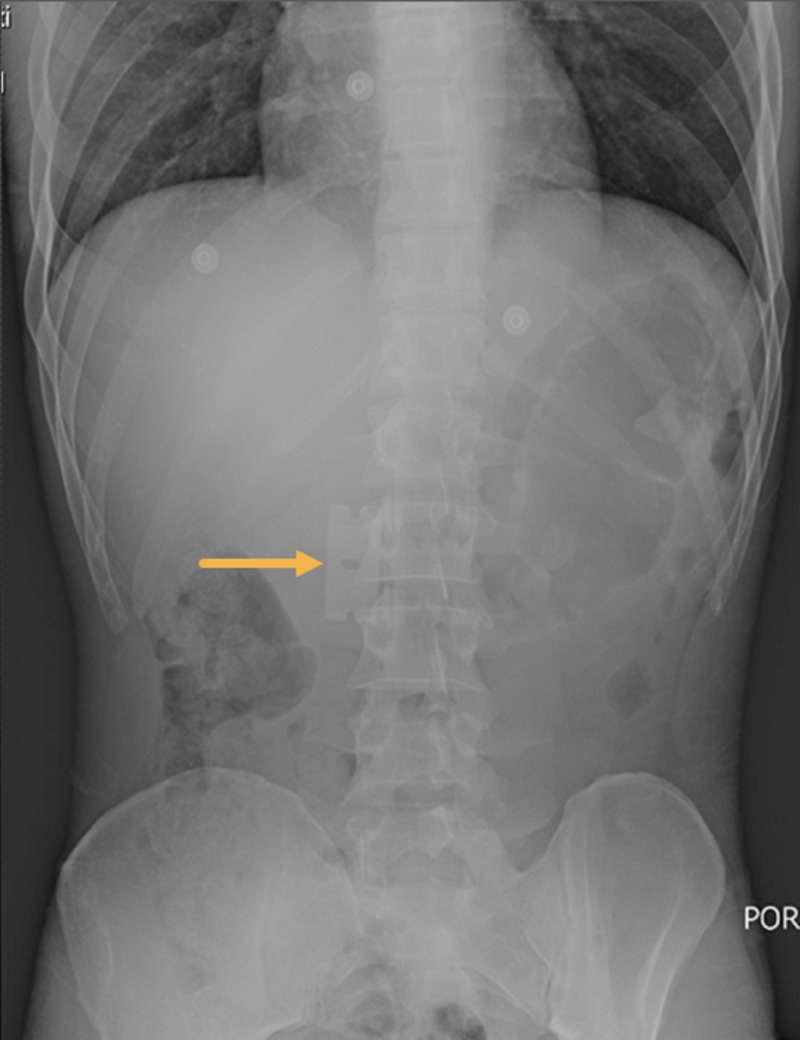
Plain abdominal radiograph showing a razor blade overlying L2 vertebral body likely in the duodenum, with no pneumoperitoneum. Arrow indicates the foreign body.
